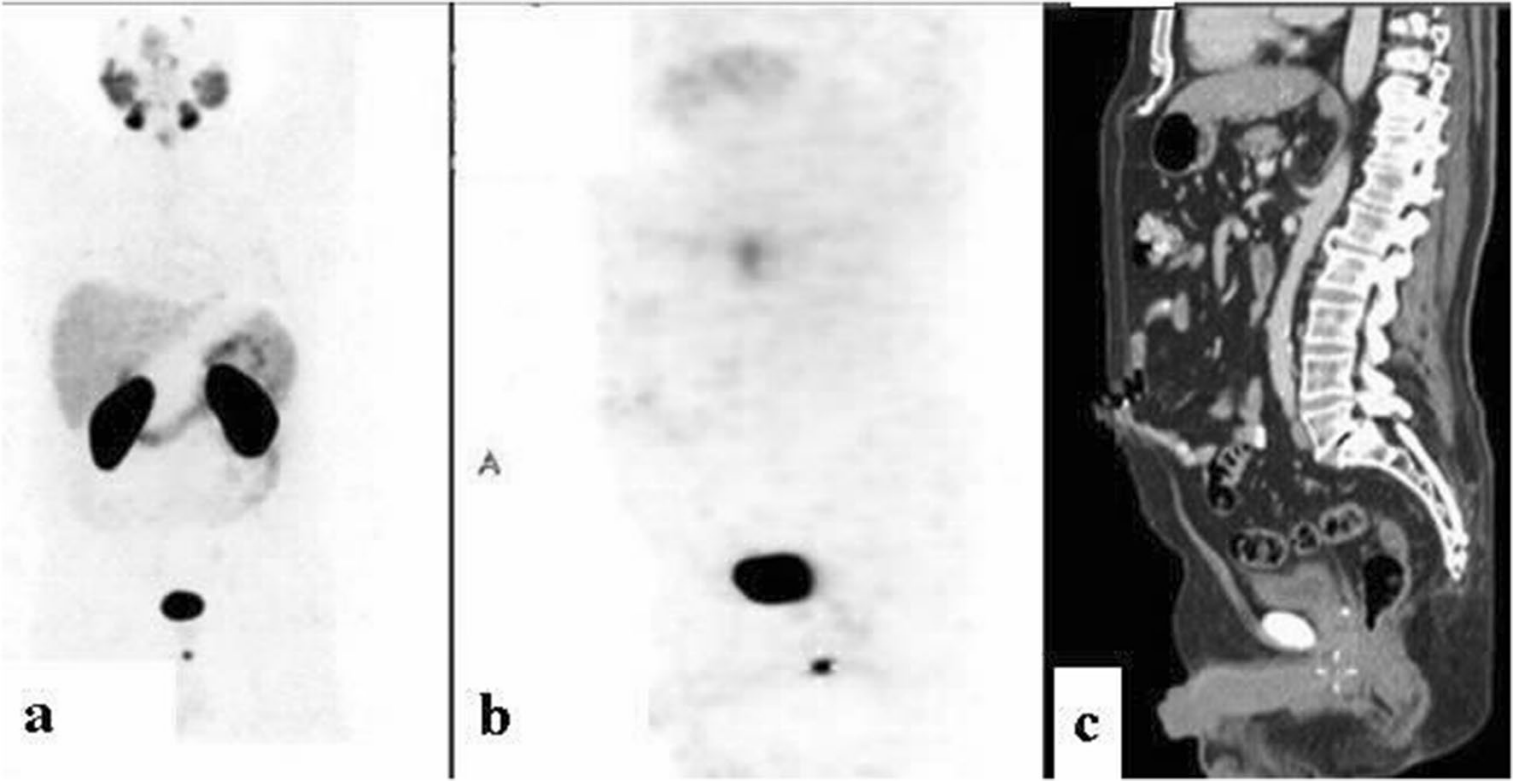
Ga-68 PSMA PET/CT: maximum intensity projection (MIP) (a) representative PET (b) and CT (c) axial slices demonstrating focally increased uptake in lesions at the root of the penis. A 62-year-old man with metastatic adenocarcinoma of the prostate with perineural invasion, who had not undergone prior prostatectomy, with a Gleason’s score of 7 and PSA of 76 (patient #6, Table 1).
